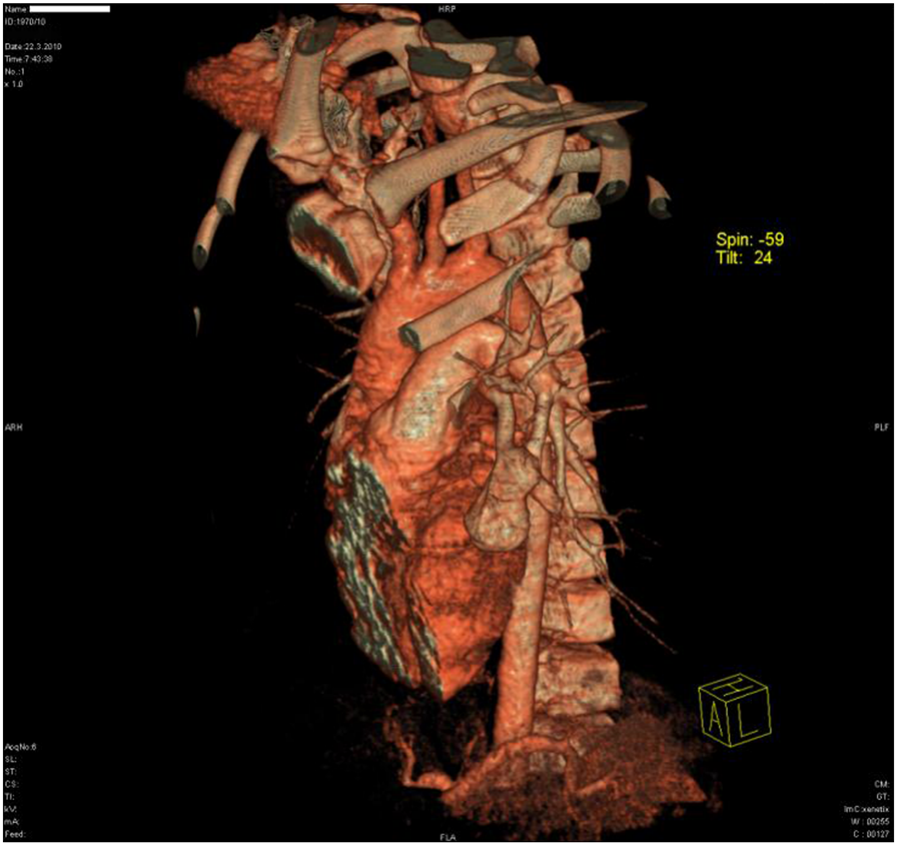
Three-dimensional computed tomography angiography revealed a 35-mm vascular malformation within the perihilar left upper lobe with a single feeding artery originating from the pulmonary artery and a single draining vein.
